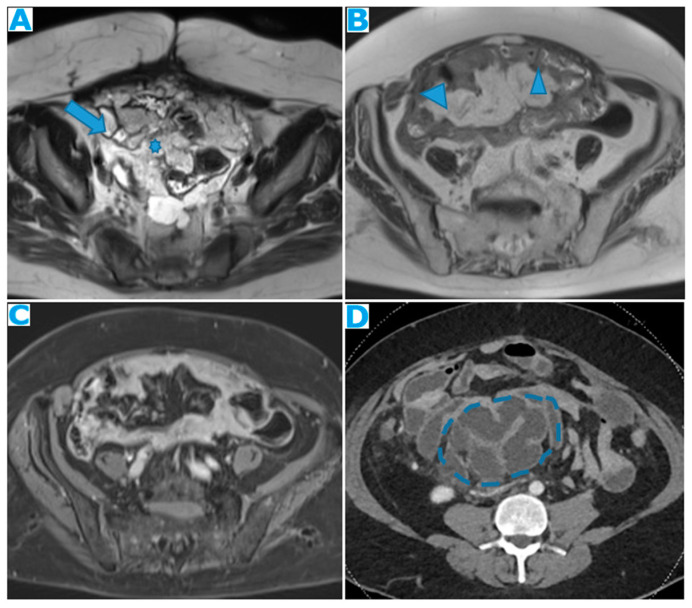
Axial T2WI (A). PC from mucinous adenocarcinoma of the urachus: notice the hyperintense mesenteric deposits (*) (signal due to mucin content) and how the SB loops appear separated and angulated (arrow). Axial T2WI (B); axial CE portal phase FST1WI (C). PC from breast carcinoma: mesenteric deposits (arrowheads on (B)) make SB loops appear thickened and separated. Notice how deposits are more conspicuous in T2WI due to its high tissue contrast. Axial CE-CT (D). PC from ovarian carcinoma: clustered small bowel loops as the end point of mesenteric seeding.
